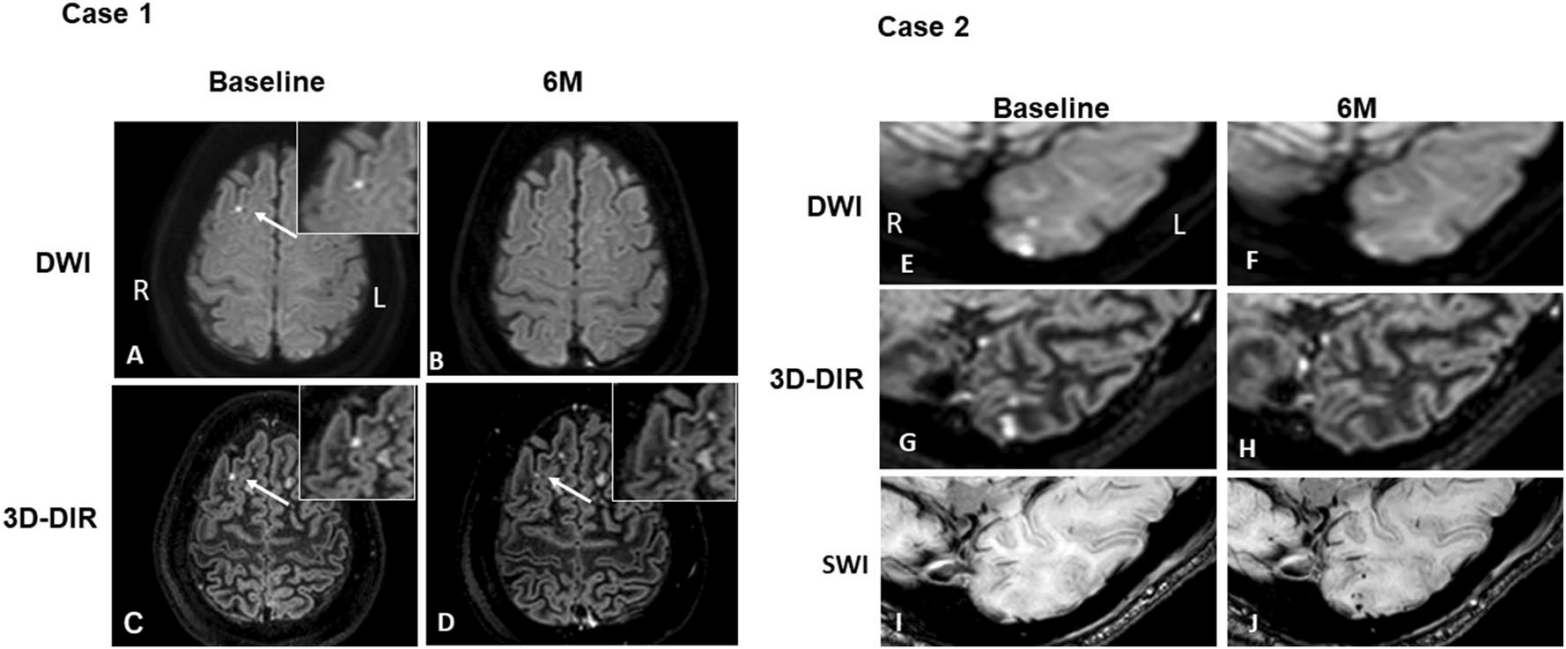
Brain MR images. Case 1: Brain MR images of a female patient. DWI (A, B), 3D-DIR findings at baseline (C) and after 6 months (D). An embolic infarct was detected by DWI and 3D-DIR after ablation. In follow-up MRI, the lesion was still detected by 3D-DIR. Case 2: Brain MR images of a male patient. DWI (E, F), 3D-DIR (G, H), SWI (I, J) findings at baseline (left) and after 6 months (right). The embolic microinfarctions detected by DWI and 3D-DIR after ablation disappeared at follow-up, but SWI detected de novo microbleeds exactly at the same location where the infarcts were detected at baseline. *DWI* Diffusion-weighted imaging, *3D-DIR* 3-Dimensional double inversion recovery, *SWI* Susceptibility-weighted imaging.
